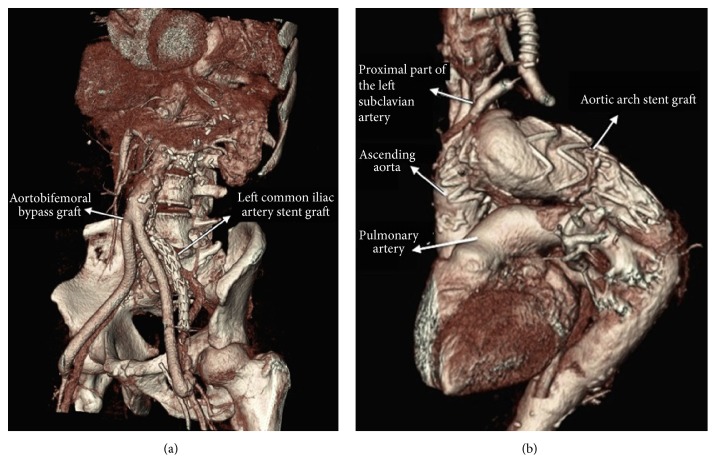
(a) Postoperative control CT angiography image of the stent graft which was applied to the left common iliac artery and the ABF. (b) Postoperative CT angiography view of the stent graft which was used in TEVAR procedure.
